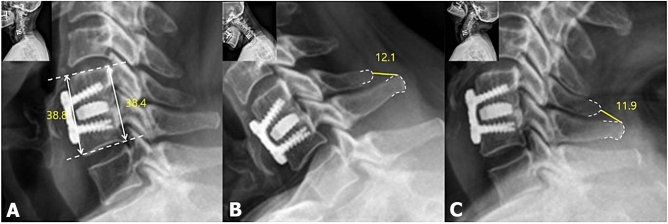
12 months postoperative dynamic lateral radiograph of a 66 years-old woman. Length of anterior and posterior border of fusion segment was measured (A). If there is 3 mm or more shorten is calculated in difference with mean of anterior or posterior border between preoperative and postoperative 12 months, defined as subsidence. Inter-spinous motion (ISM) is measured between most prominent point of spinous process within fusion segment in (B) Flexion and (C) Extension view. if there is > 1 mm difference, was defined as pseudoarthrosis.
